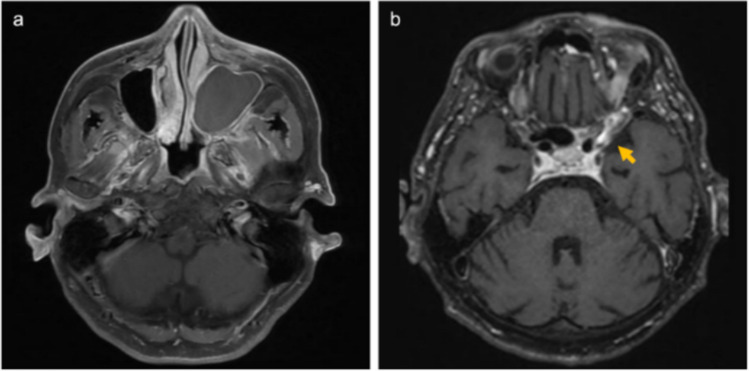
An abnormal signal filling the left maxillary sinus shows hyperintensity on T1-weighted images without appreciable contrast enhancement (a). Dural thickening extends from the left cavernous sinus to the middle cranial fossa (b, arrow)
